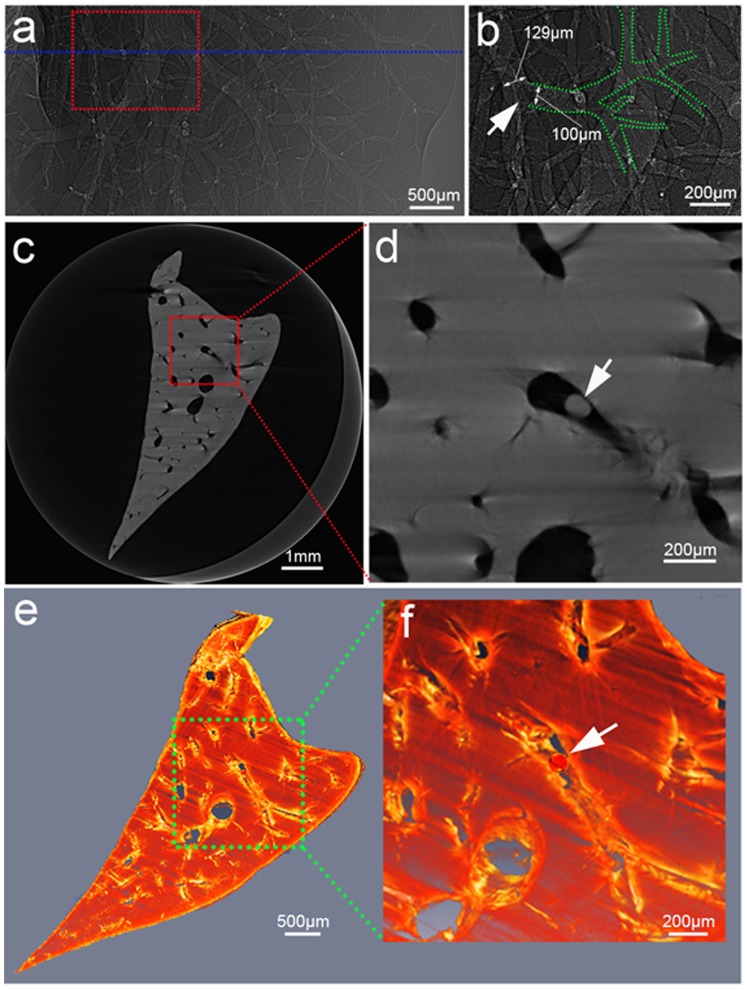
Phase contrast CT imaging of FCM-embolized dehydrated liver. Projection image (a) and reconstructed views (c,e) visibly show the FCM in the embolized portal vein. (b), (d) and (f) are magnified images of the region in a dotted box in (a), (c) and (e), respectively. (b) An about 100-µm portal vein and its branches (outlined in green) are clearly displayed to be embolized by a 100-µm FCM. (c) is the axial slice across the blue line in (a). (f) The 3D rendering shows spatial distribution of the microsphere inside the liver. Arrows indicate FCM. Images were obtained at the energy of 19 keV with the sample-to-detector distance of 60 cm. The pixel size was 3.7 µm×3.7 µm; the exposure time was 1 s.
